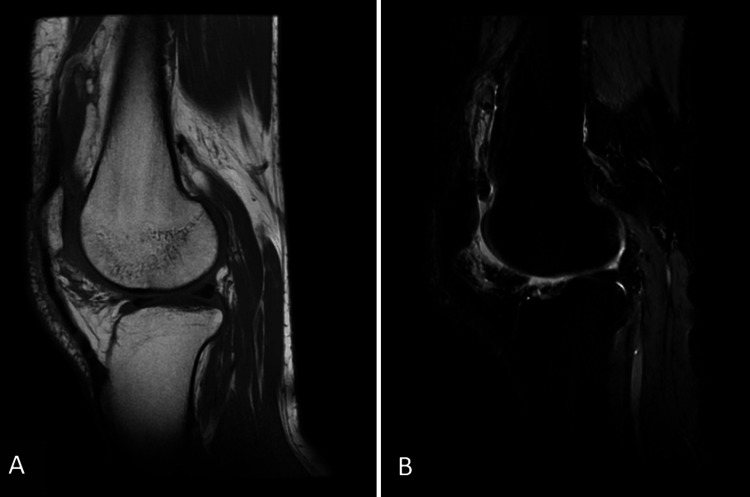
Sagittal MRI of the knee. (A) T1-weighted image demonstrating frond-like synovial proliferation with high signal intensity identical to subcutaneous fat. (B) Sagittal proton density fat-suppressed image showing complete signal suppression of the synovial lesions, confirming their fatty nature.
